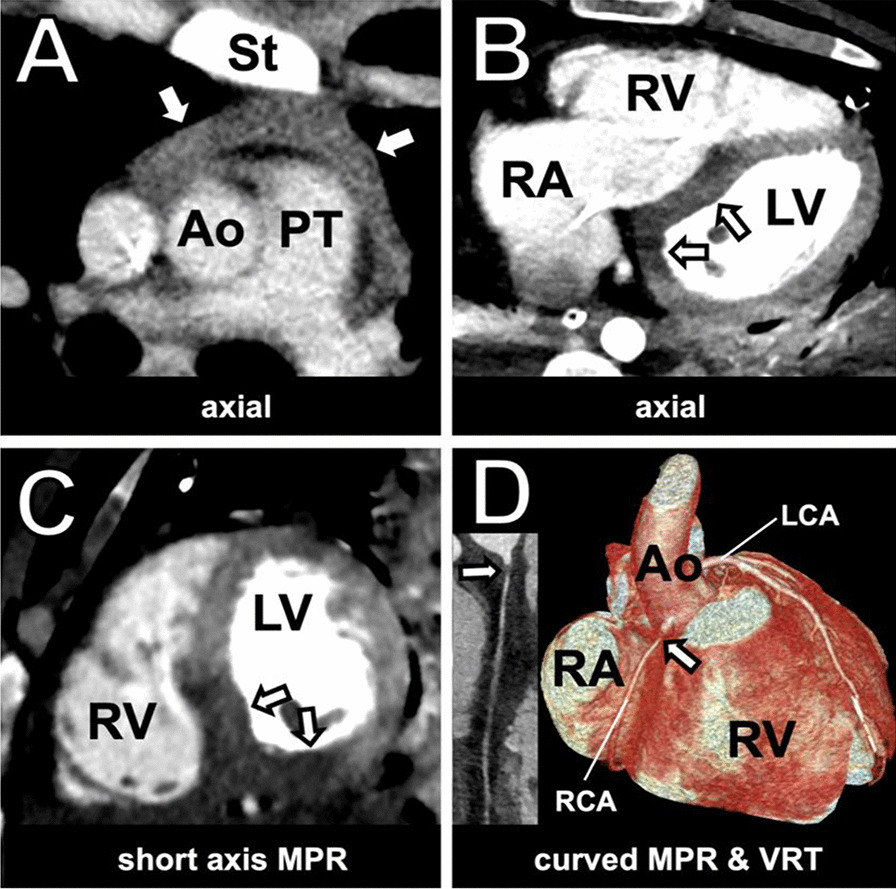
Contrast-enhanced CT and CTCA showing pericardial effusion, right heart strain and proximal RCA dissection. A Contrast-enhanced CT showed a progressive pericardial effusion (white arrows). B, C Massive right heart strain and inferoseptal myocardial infarction (white arrows). D Coronary CT angiography revealed a short proximal discontinuation (white arrows) of the RCA, highly suggestive of dissection
